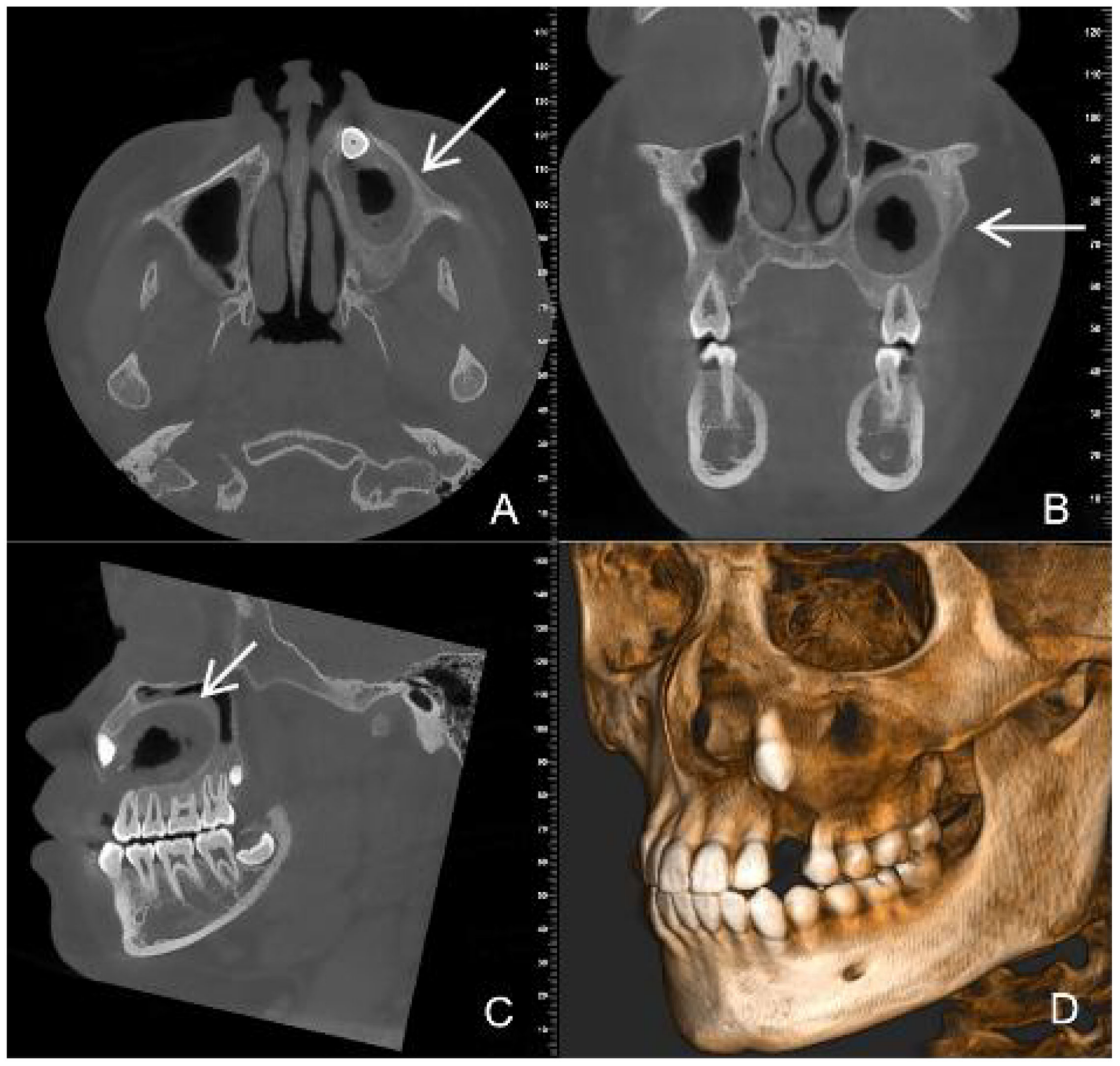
CBCT images six months after decompression surgery (A) (axial section) (B) (coronal position) (C) (sagittal position) (D) (three-dimensional reconstruction) show that the lesion size has become smaller and the bone repair area (white arrow) more obvious.
